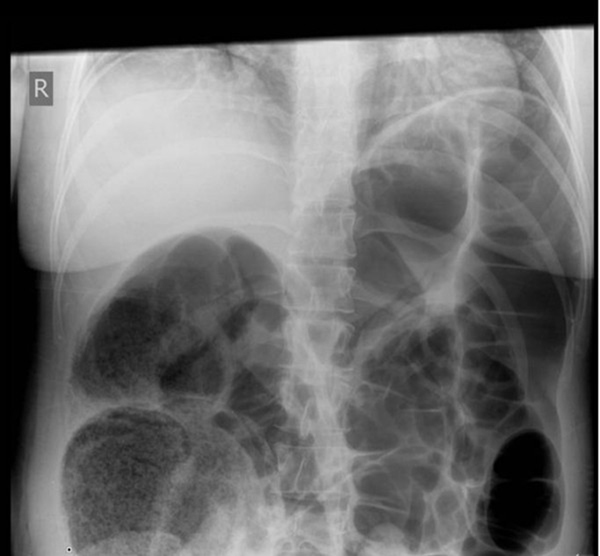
Plain supine abdominal radiograph showing widespread colonic dilatation.
